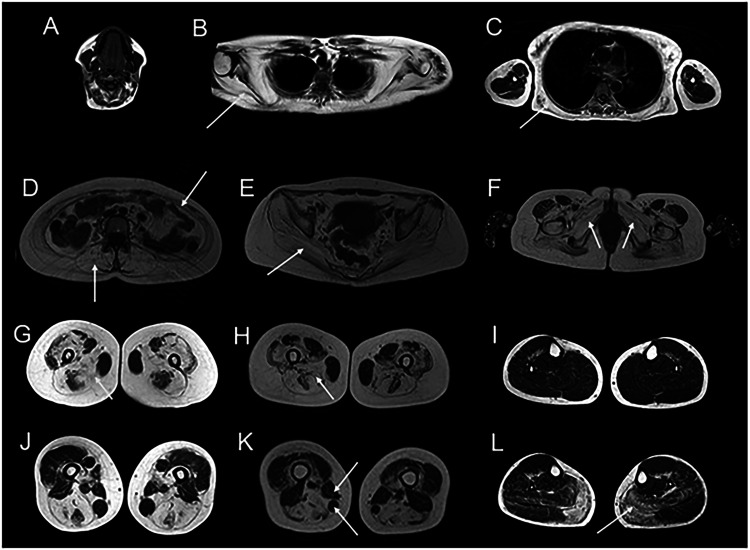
Findings on muscle MRI studies in sarcoglycanopathies.
